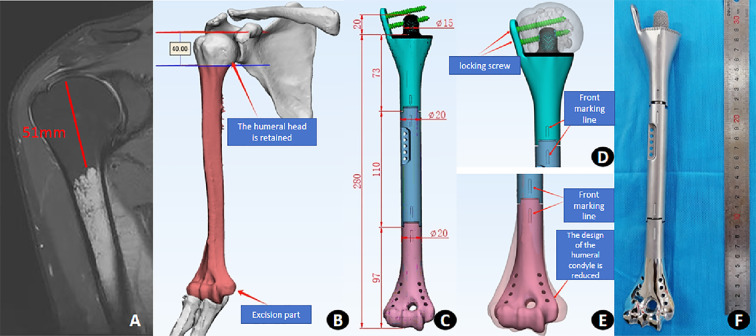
Preoperative imaging and prosthesis design. (A) Preoperative MRI demonstrated that the proximal 5 cm of the humerus was free of tumor invasion. (B) After CT scanning, the patient’s right humerus was three-dimensionally reconstructed. During surgery, a 4-cm margin of healthy bone at the proximal humerus will be preserved. The red-marked area indicates the pathological tissue to be resected. (C) The semi-elbow joint humeral prosthesis is designed in three structural segments: proximal, middle, and distal. (D) The proximal 4 cm of the humeral head is preserved, and the prosthesis is fixed using a lateral plate and a short intramedullary stem. Alignment marks on each segment ensure correct orientation and prevent rotational misalignment during implantation. (E) The distal component of the prosthesis is designed to be 15% smaller than the native humeral condyle and incorporates dedicated channels for fixation of surrounding soft tissues. (F) Personalized prosthetic devices.
